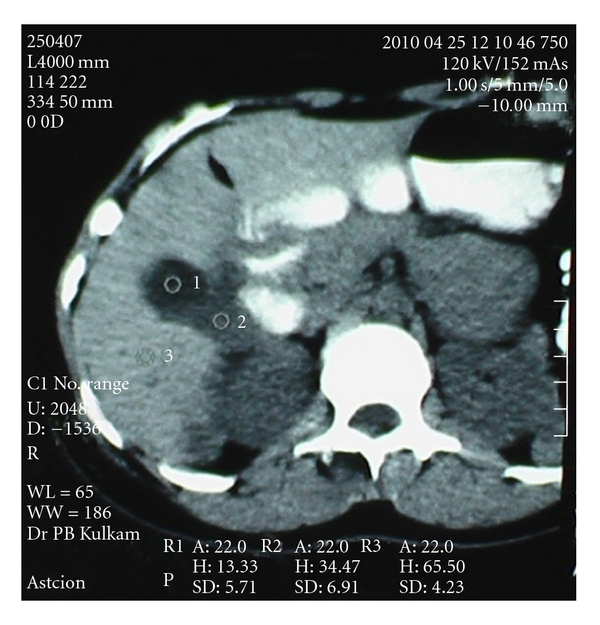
CT Scan of liver hydatid cysts.
